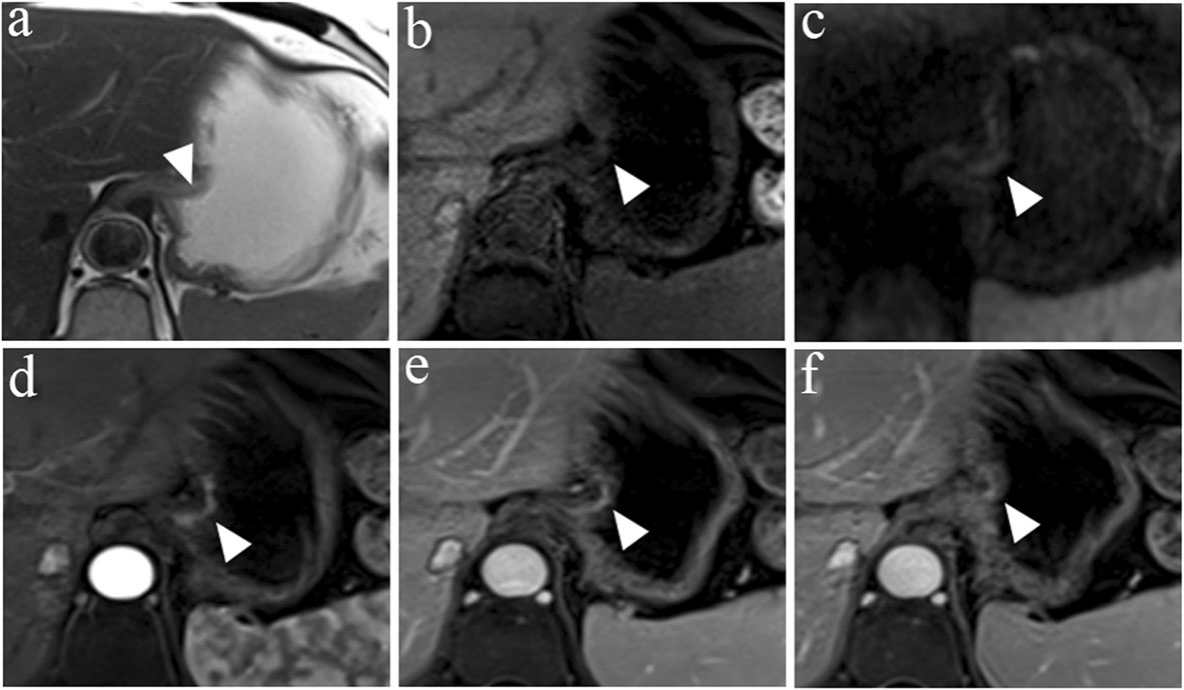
A 68-year-old woman with esophagogastric junction cancer was pathologically diagnosed using HR MRI as having stage T1b, Sierwert II. a The axial T2W image shows partial thickening of the cardia wall with a decreased signal (arrow). b The axial diffusion-weighted image with b = 800 s/mm^2^ shows restricted diffusion in the cardia. c The lesion was not clearly shown on the axial T1W image. d Axial and arterial phases of a contrast-enhanced image show significant enhancement of the lesion (arrow). e Persistent enhancement of the lesion was observed on the enhanced and venous phases of T1W images. f The lesion and mucosal tissue had similar degrees of enhancement on the delayed phase of the T1W image
